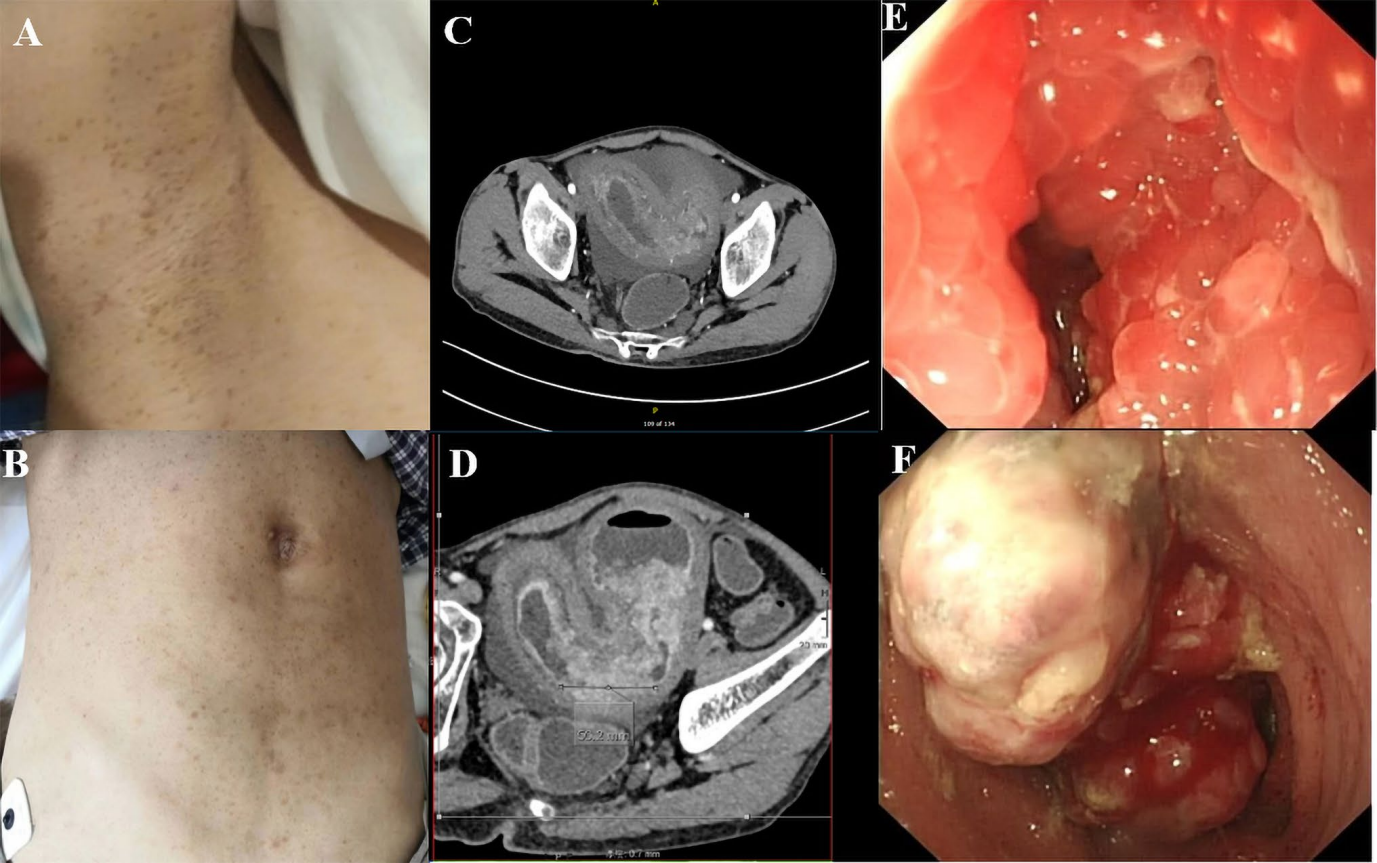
(A, B) Physical examination revealed freckling at axillary and diffused café‐au‐lait macules on skin. (C, D) Abdominal computed tomography showed marked thickening and mild enhancement in the ileal mucosa and mesentery with multiple enlarged lymph nodes. (E, F) Enteroscopy showed multiple nodular bulges in the circumferential mucosa with apparent stenosis in the lower ileum and multiple nodular protrusions covering about half of the lumen in the distal ileum.
